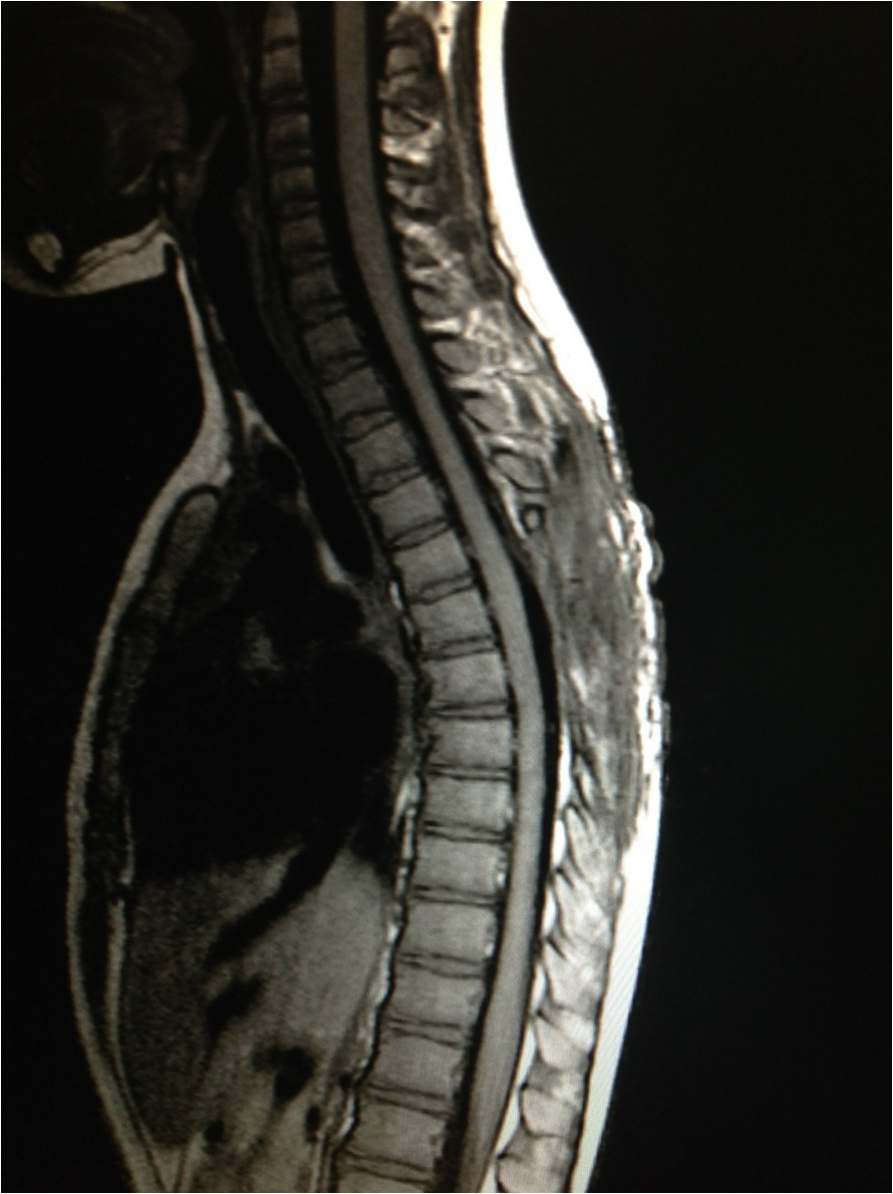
Postoperative magnetic resonance imaging at 1 year. Sagittal T1-weighted magnetic resonance imaging at one-year follow-up showed there was no recurrence of the tumor.
